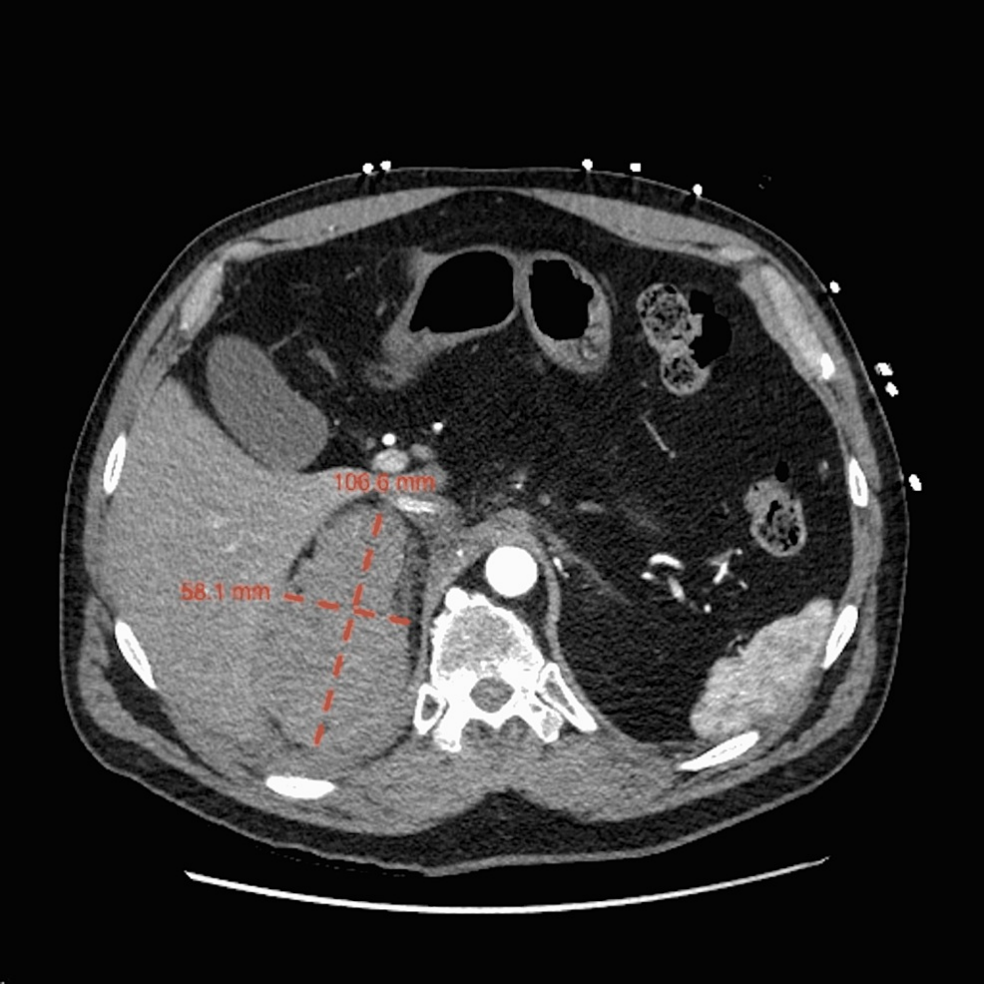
Axial CT image demonstrating a right retroperitoneal hematoma in the region of the right adrenal gland measuring 106.6 mm x 58.1 mm. There are areas of enhancement on arterial phase imaging, which represent congestion of a draining vein or delayed active extravasation.
